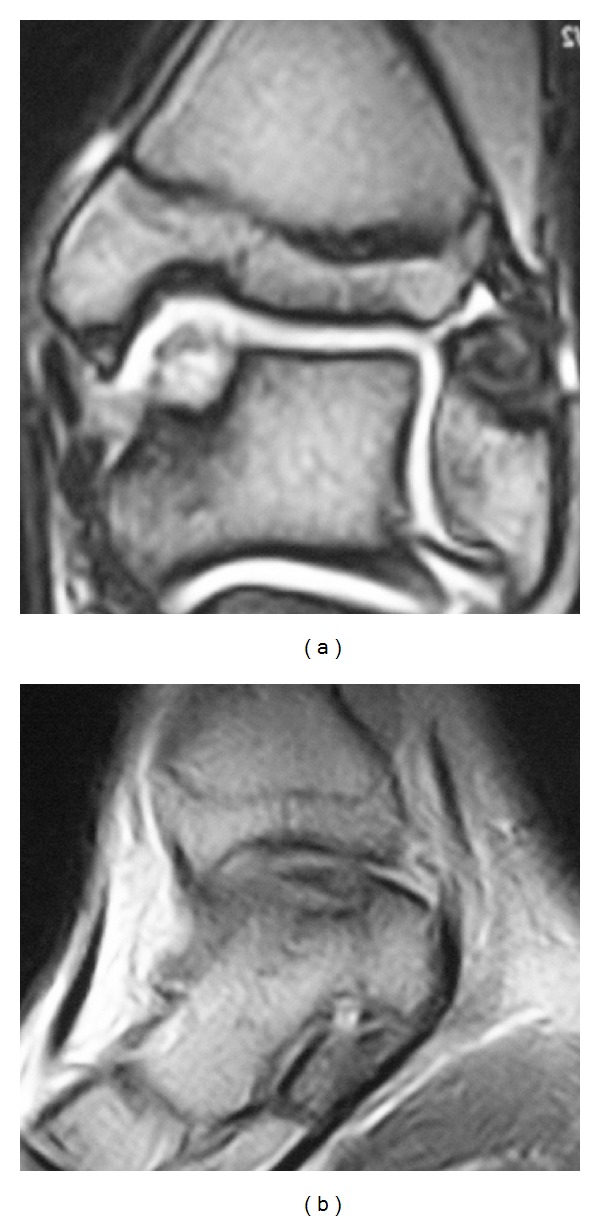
Preoperative coronal (a) and sagittal (b) MRI views before the first operation, showing a IIA type lesion, according to Giannini's classification.
